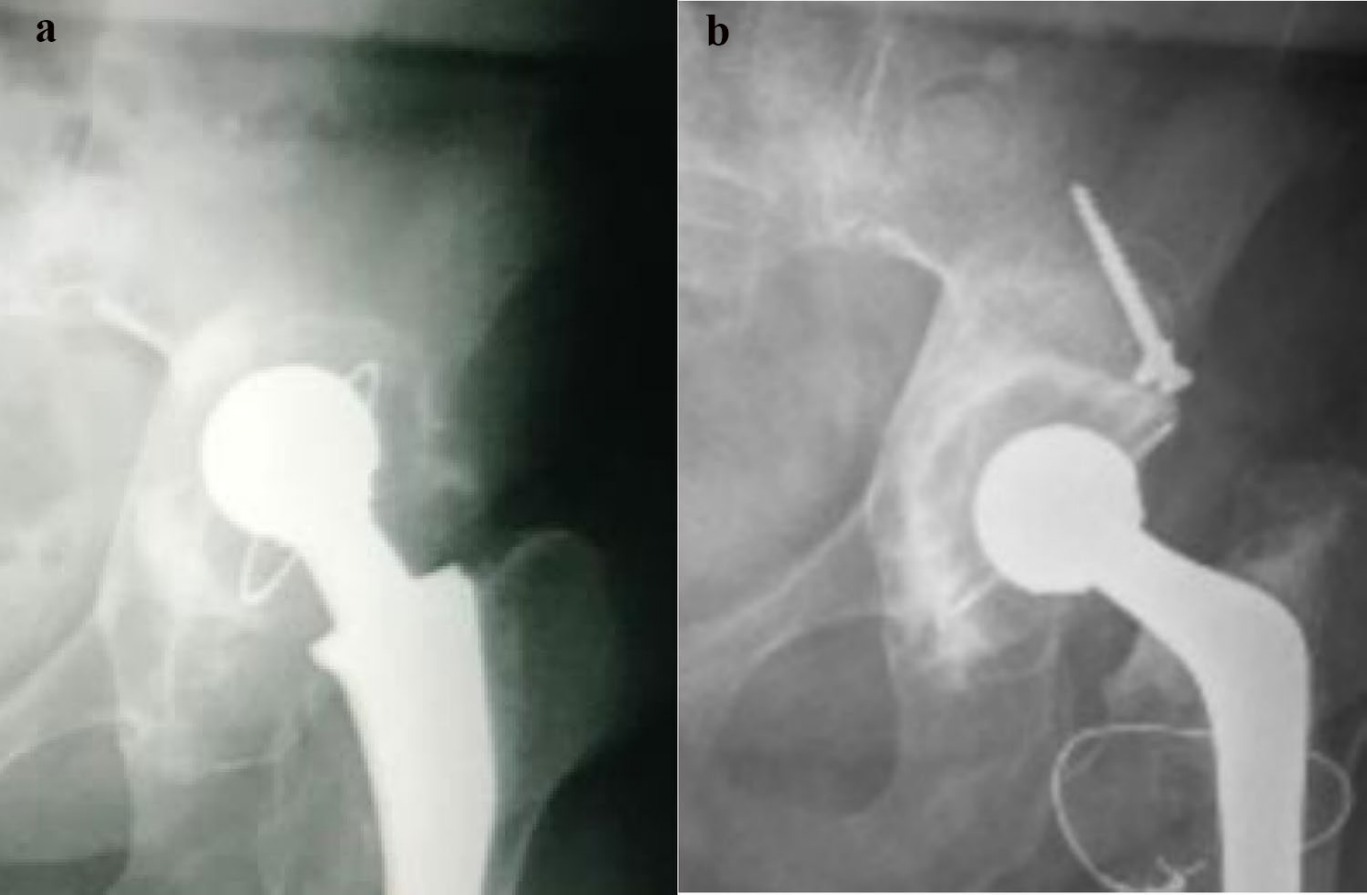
A Paprosky type 3A acetabular defect; B postoperative radiograph at 43 months follow-up. Source: the Authors
